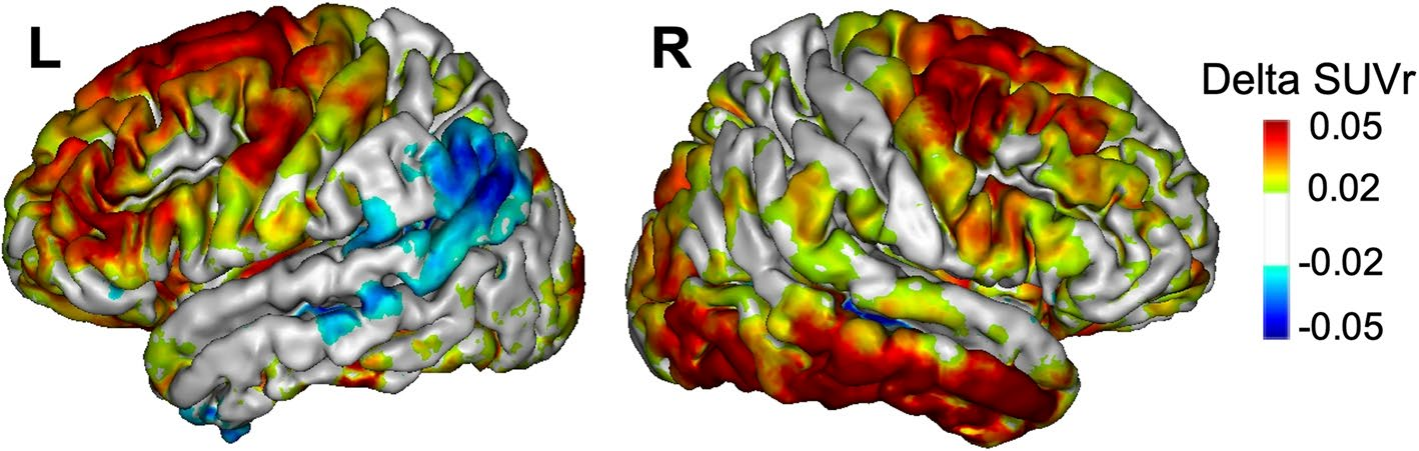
Progression of tau SUVr values in AD patients. Average annualized differences in SUVr between Tau1 and Tau2 (Tau2-Tau1) in AD patients (projection of the left (L) and right (R) hemispheres on the 3D MRI MNI template)
